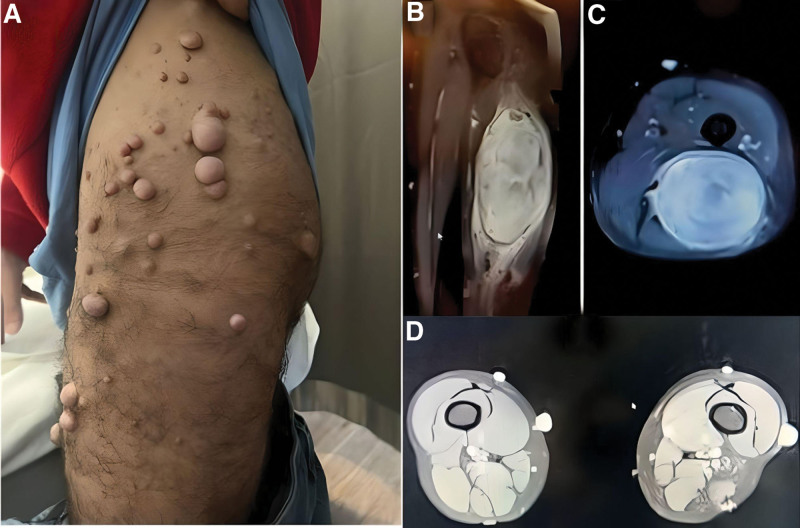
(A) Notice the multiple neurofibromas on the lateral aspect of the left thigh. (B) Contrast-enhanced MRI showed a 7.5*8.5*14.5 cm solid mass (schwannoma) extending along the sciatic nerve; it was mildly hyperintense on T1-weighted image. (C) Notice the tumor markedly contrasted and hyperintense on T2-weighted axial image on post-contrast sequence. (D) MRI follow-up, T1-weighted images, showed no recurrence. MRI = magnetic resonance imaging.
